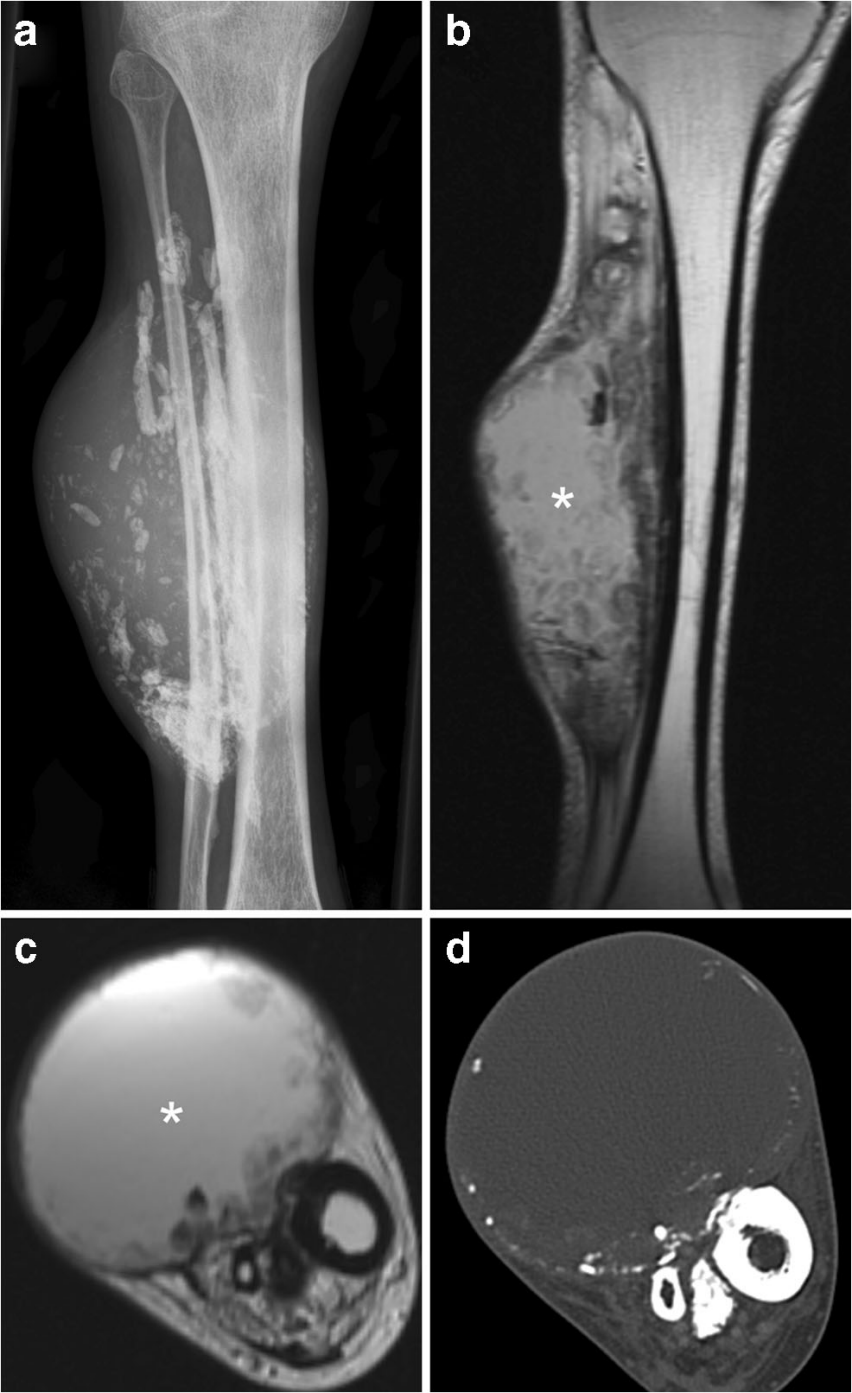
A 72-year-old man with calcific myonecrosis of the anterior compartment of the right leg. a Radiography shows a soft tissue mass with extensive calcifications. b, c Axial (a) and coronal (b) proton-density weighed images shows the mass to be located within the anterior compartment of the right lower leg, with a liquid-appearing center. d Axial CT shows the typical peripheral calcifications
